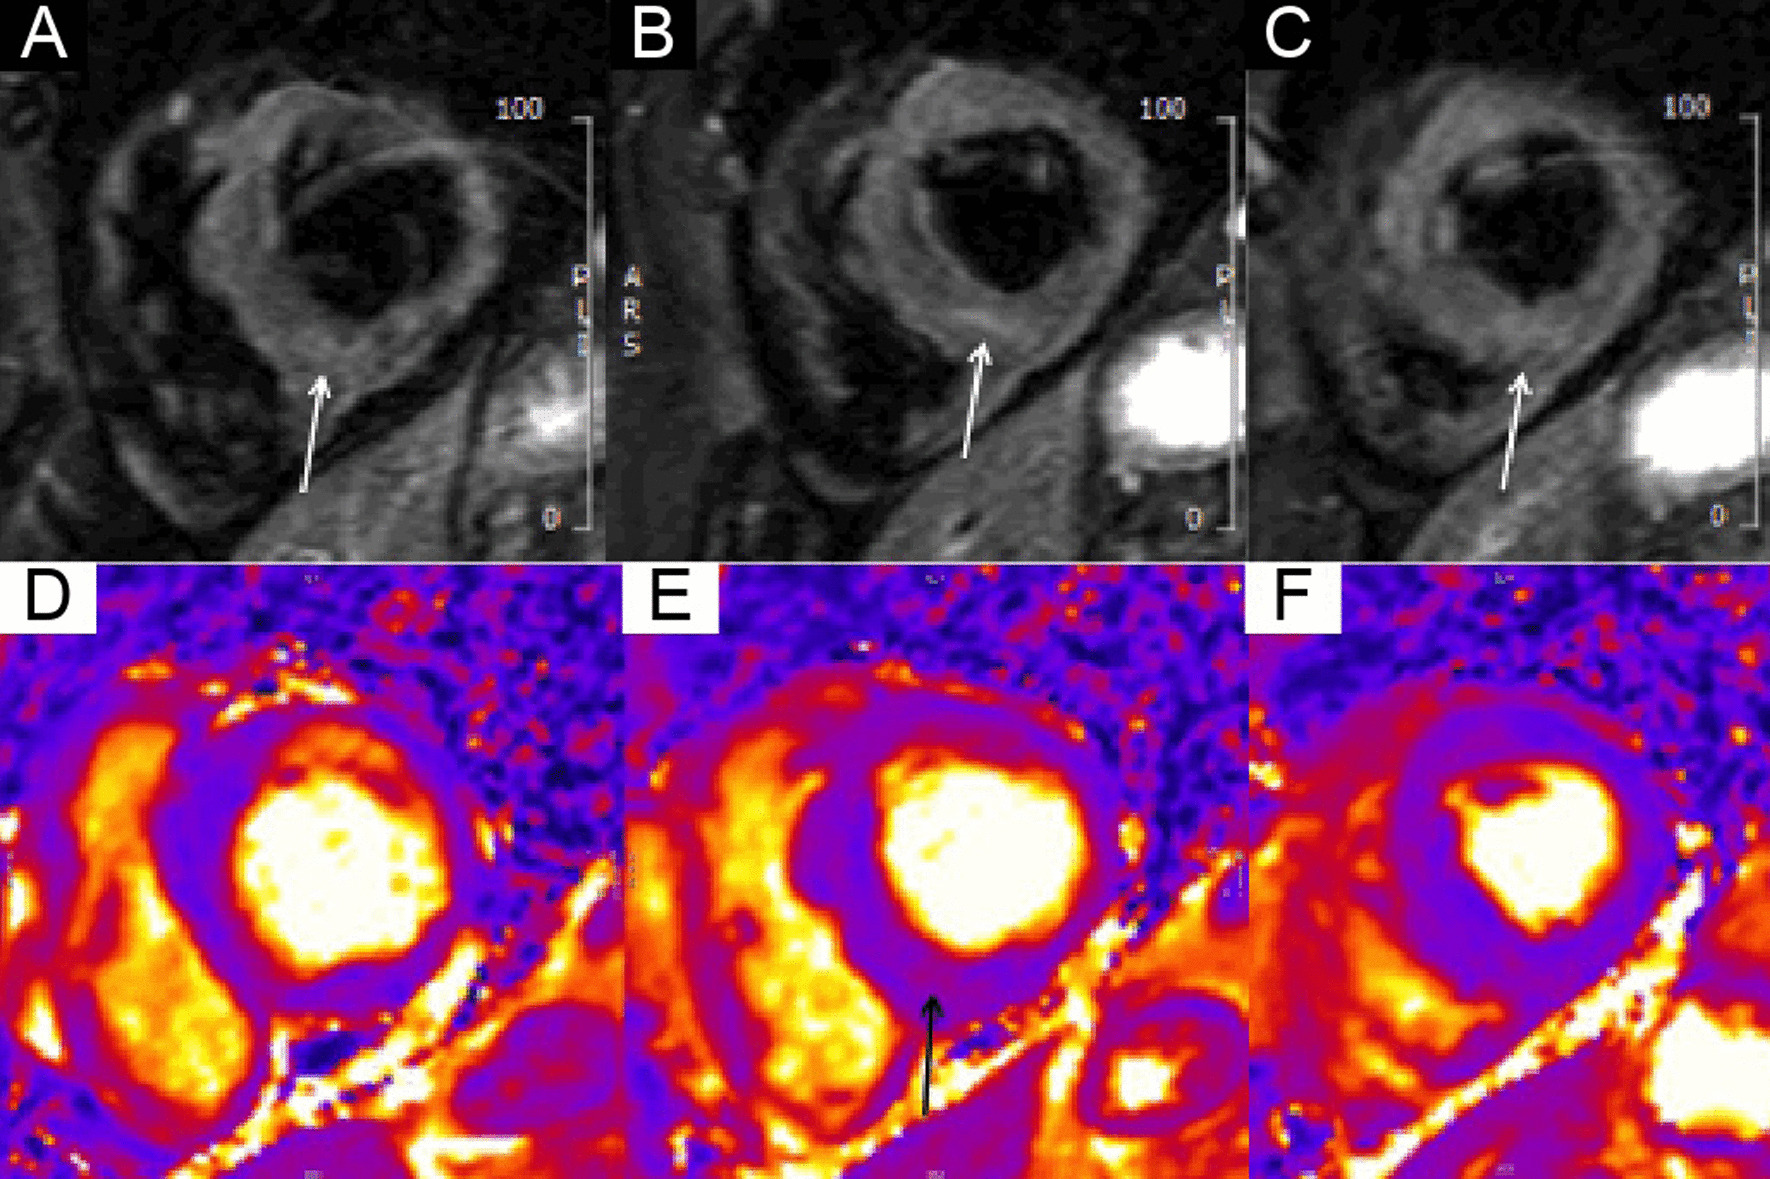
Case 2. Figure 2. Basal short axis T2-weighted sequences (A–C). Basal short axis T2-mapping images (D–F). The T2-weighted images show mid-wall increased signal intensity in the basal inferoseptum, consistent with myocardial oedema (white arrows). The T2-mapping images show increased T2 values in the basal inferoseptum (black arrow)
